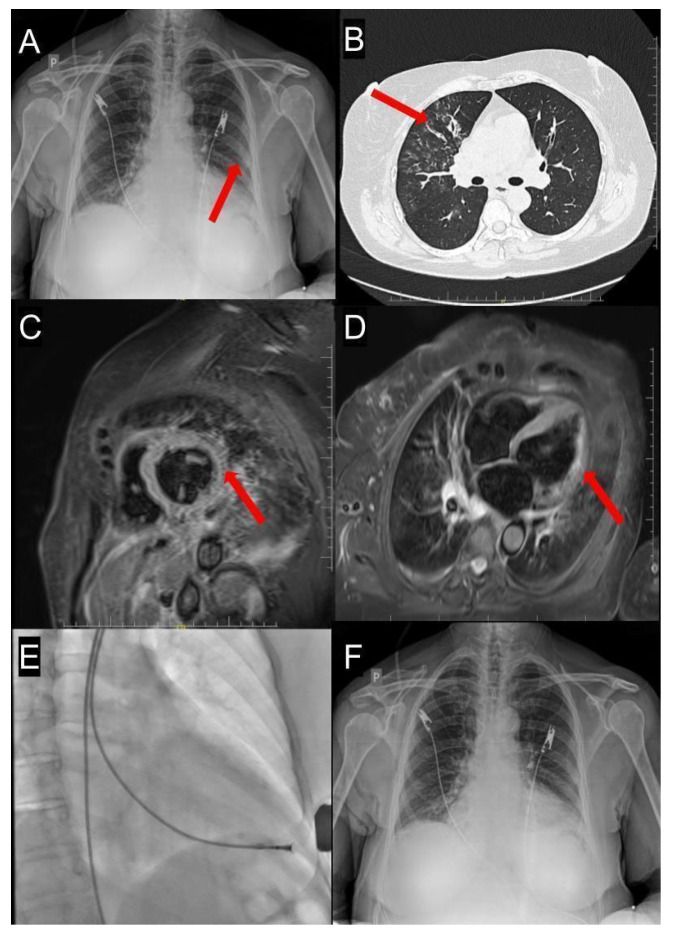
(A) Initial chest X-ray showing inflammatory changes consistent with pneumonia (indicated by the red arrow). (B) High-resolution chest CT (HRCT) showing bilateral inflammatory changes with a characteristic “tree-in-bud” pattern (marked by the red arrow). (C) Cardiac magnetic resonance (CMR) in a SAX view demonstrating generalized myocardial edema with late gadolinium enhancement (LGE) highlighted by the red arrow. (D) CMR in a four-chamber view demonstrating generalized myocardial edema with late gadolinium enhancement (LGE) highlighted by the red arrow. (E) Fluoroscopic image of a left ventricular muscle biopsy from the apical region, performed under echocardiographic guidance. (F) Follow-up chest X-ray at discharge showing a gradual resolution of the inflammatory changes.
